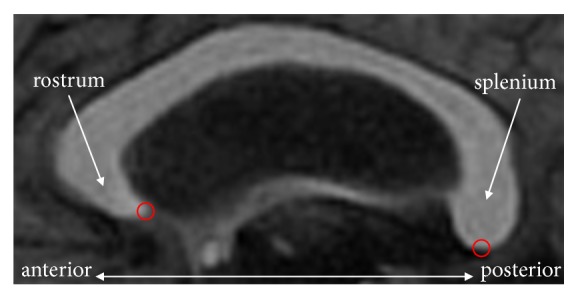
Endpoints (centers of the red circles) of the CC in a mid-sagittal MR image.
